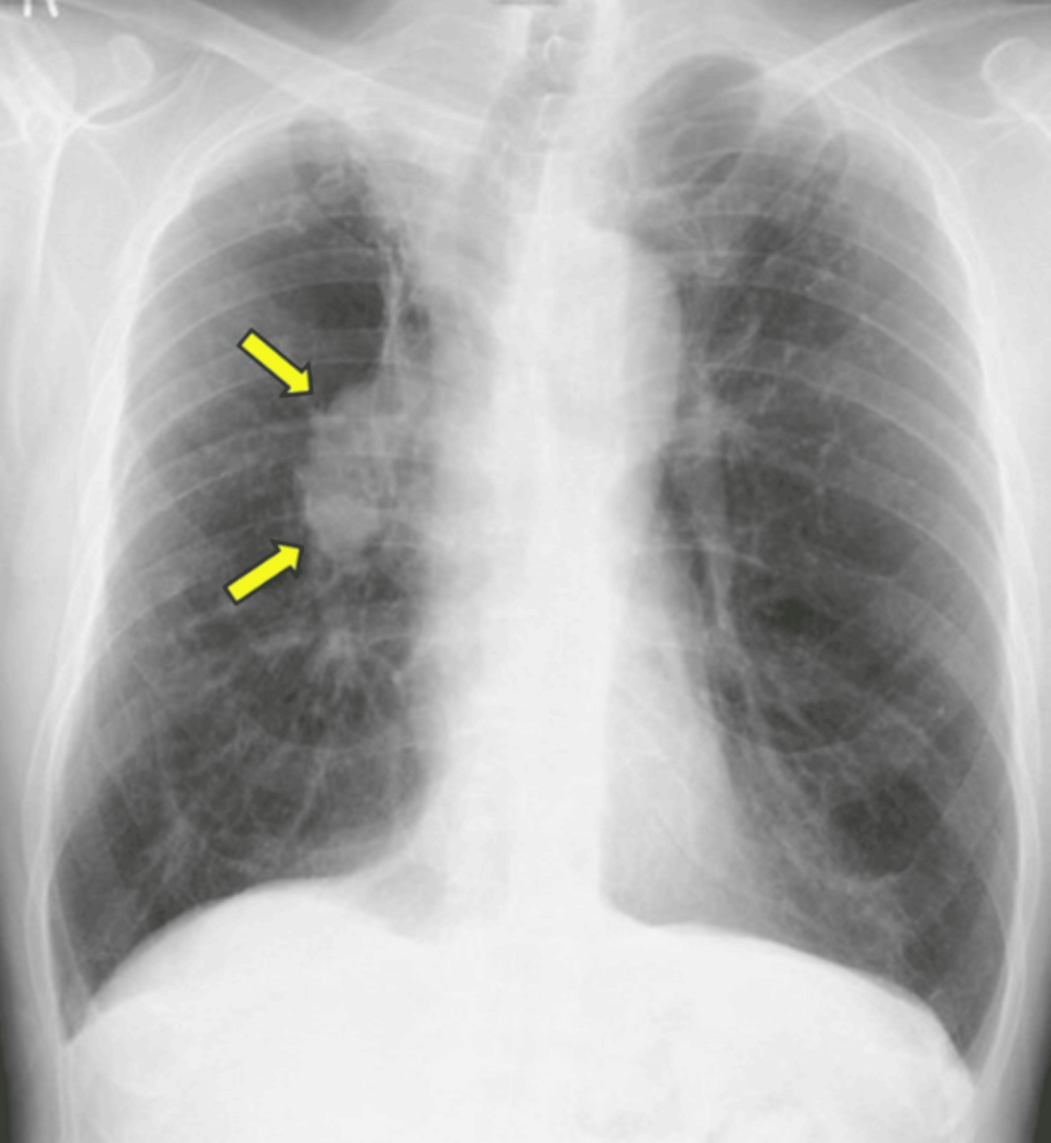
Chest radiograph showing a 60 × 35 mm mass (yellow arrows) in the right hilum
